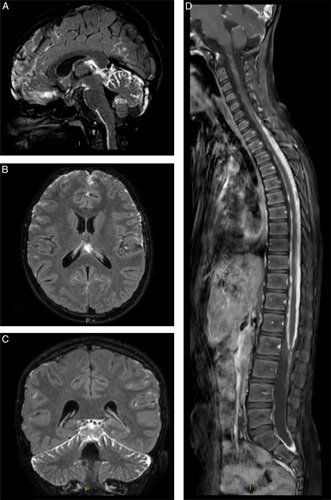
Brain and spinal MRI. Postcontrast T2-weighted 3D FLAIR fat-suppressed sequences, reconstructed in the sagittal (A), axial (B), and coronal (C) planes. MRI scan demonstrates diffuse leptomeningeal enhancement, most prominent within basal cisterna, posterior fossa, and along frontal and interhemispheric cerebral spaces. Sagittal T1-weighted postcontrast fat-suppressed image (D) shows thick leptomeningeal enhancement, predominantly along the dorsal thoracic spinal cord. MRI indicates magnetic resonance imaging.
